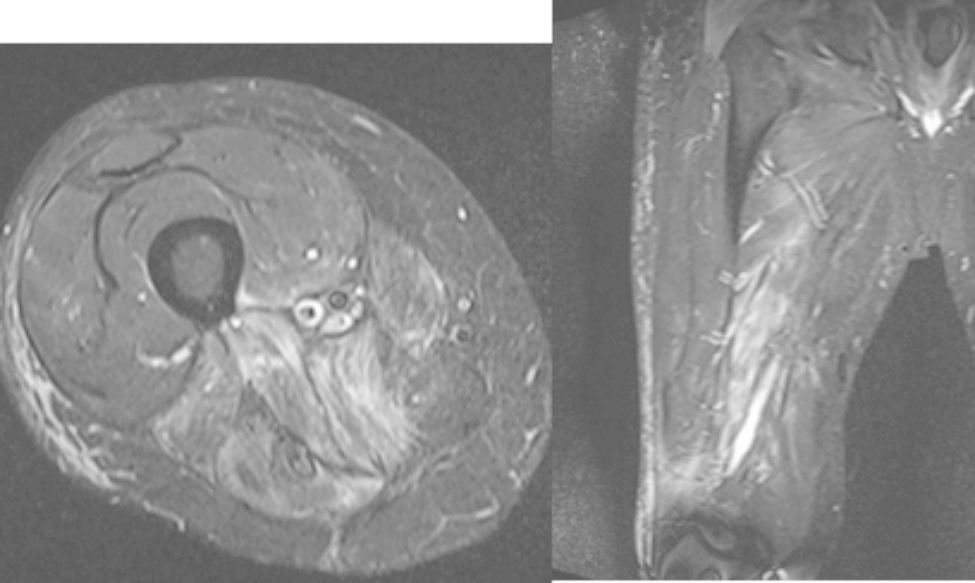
Postoperative T2-weighted MRI (left: axial; right: coronal)
